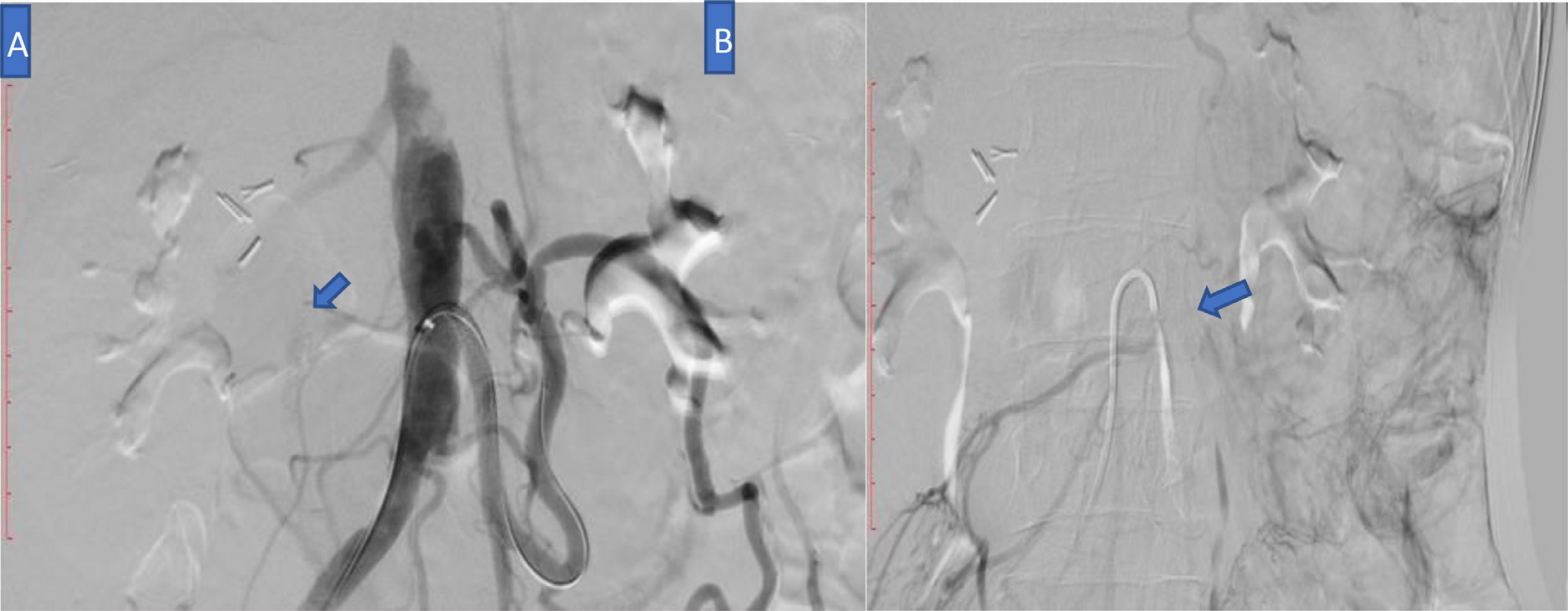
(A) IMA stenosis on the conventional radiography (B) Percutaneous transluminal angioplasty (PTA) to IMA and balloon dilatation.
